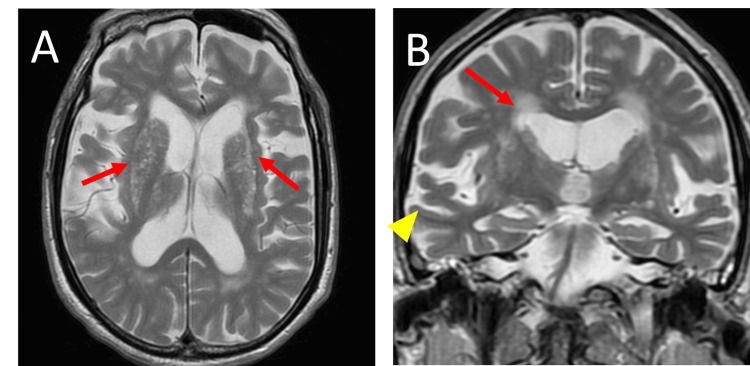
Brain magnetic resonance imaging of patient 3 (A) T2-weighted horizontal image and (B) coronal image show T2 hyperintensity (arrows) in periventricular white matter, basal ganglia, and temporal lobe atrophy (arrowhead).
